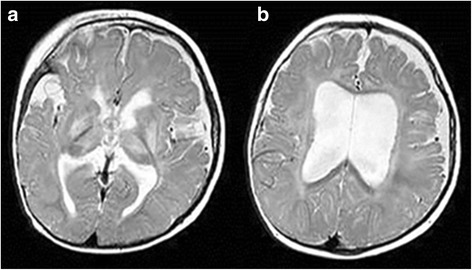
Brain MRI at one month of age. Axial T2-weighted MR image obtained at a ganglionic (a) and supraganglionic (b) level shows an increased signal intensity in the lentiform nuclei and ventrolateral thalami, in the periventricular white matter and within the subcortical U-fibers. Furthermore, the images show an enlargement of the lateral ventricles with irregular margins, fluid collection in the fronto-parietal area and a widening of the Silvian fissure
